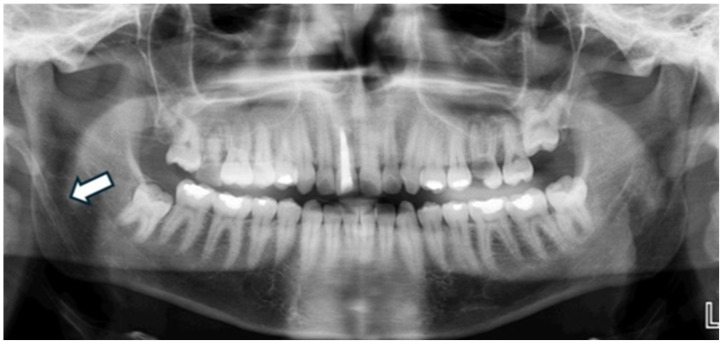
Panoramic radiograph of a 48-year-old male patient demonstrating a unilateral, elongated, and radiopaque structure extending downward from the right temporal region, indicative of styloid ligament calcification.
